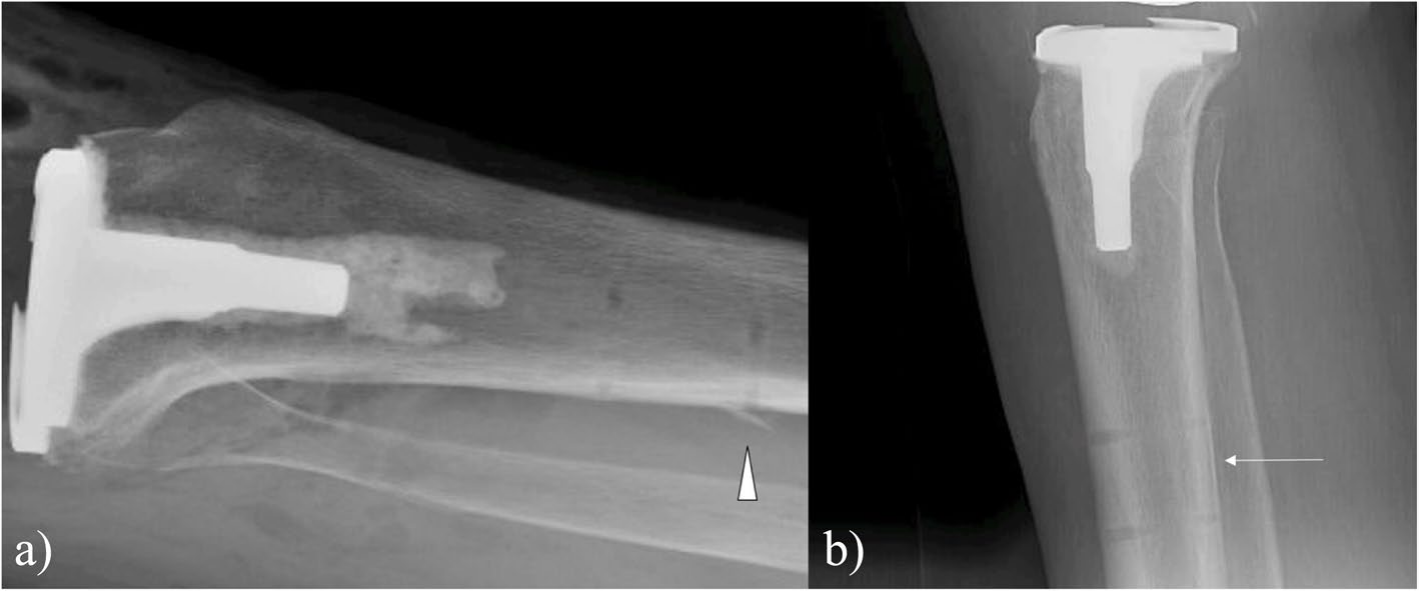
a Lateral radiograph of a 70-year-old female with an intraoperative pin site cortical fleck (arrowhead) involving the distal tibial pin site of her right knee. b Lateral radiograph of an 83-year-old female with an intraoperative nondisplaced pin-site fracture of her right tibia as evidence by linear lucency (arrow) noted along the posterior aspect of the proximal tibial shaft between both pin sites. Both cases involved the large pin diameter robotic-assisted total knee arthroplasty system
